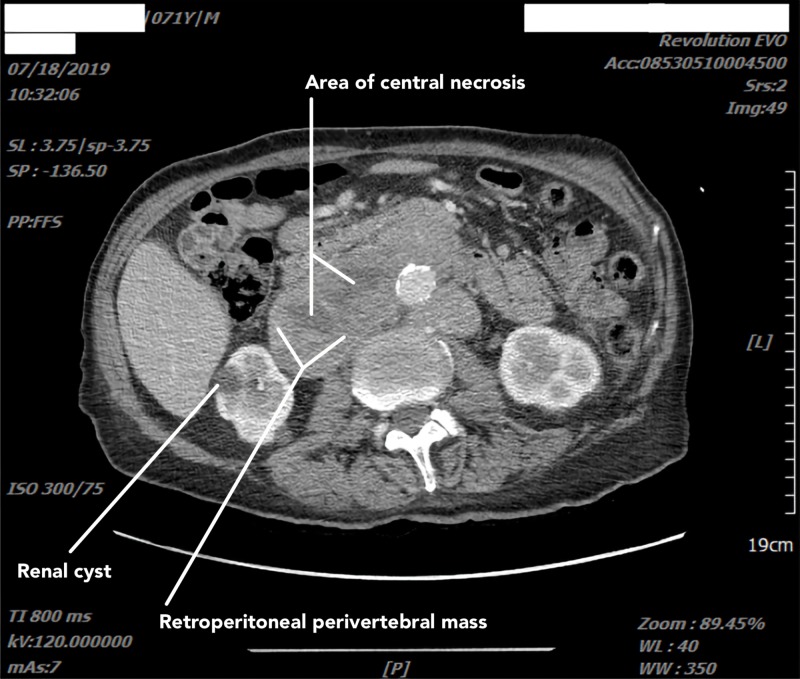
Abdominopelvic CT scan with contrast shows a retroperitoneal perivertebral mass measuring 11.9 cm in the largest dimension.
